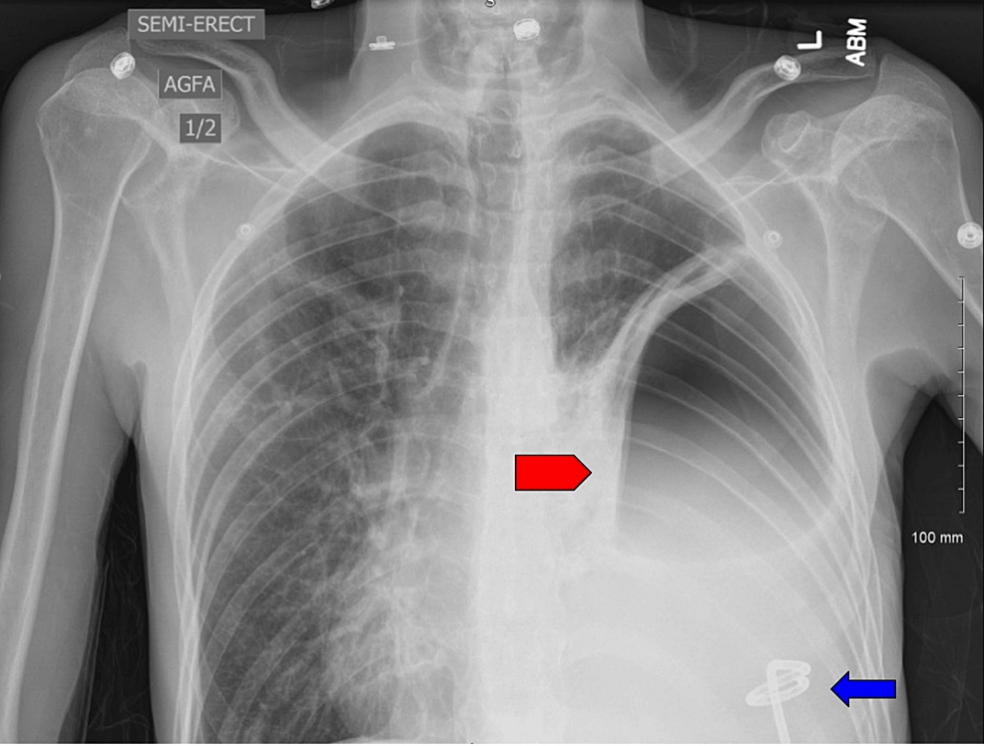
Chest X-ray on day 3 of admission Left lung base pleural catheter (blue arrow) with a loculated pneumothorax at the left lung base (red pentagon), reflecting pneumothorax ex vacuo.
